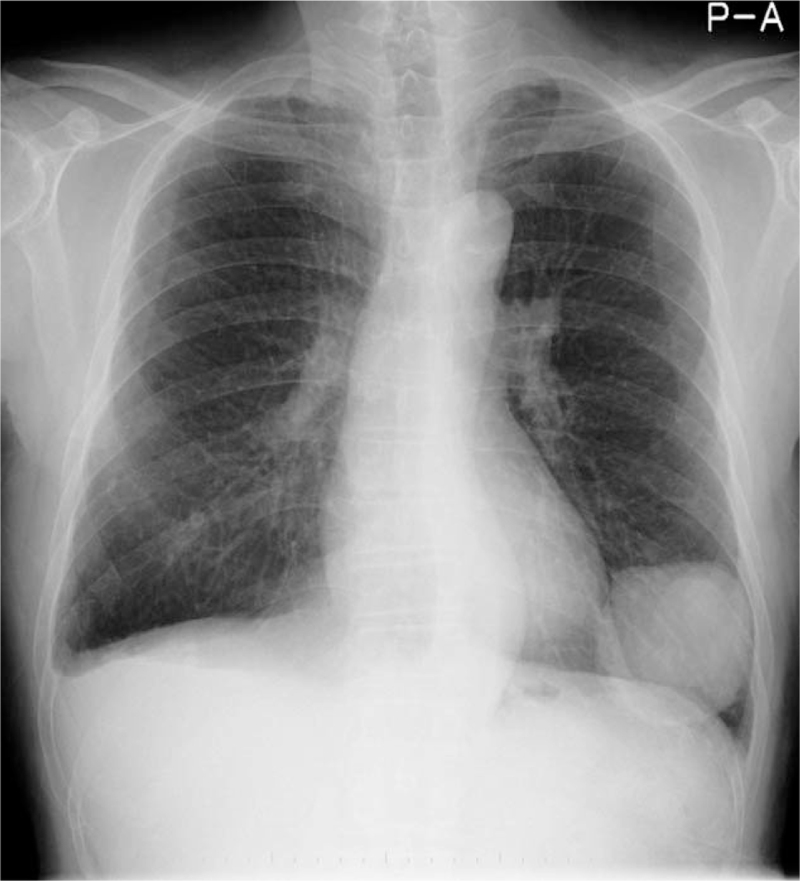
Chest radiograph showing a rounded mass shadow in the left lower lobe.
